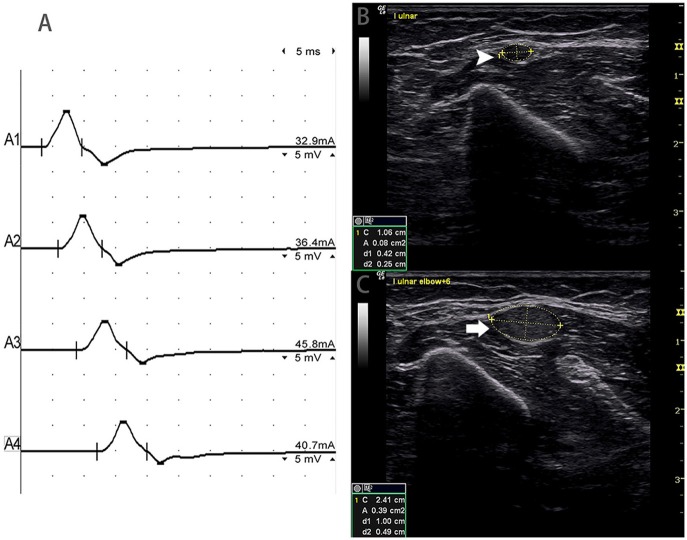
Mode 3. A 51-year-old woman with 4 years of left upper limb weakness and MRC grade 4 showed CSA enlargement without corresponding conduction block. (A) Standard segment motor nerve conduction study of the left ulnar nerve. No CB was detected. Nerve ultrasound study across the left upper arm of the ulnar nerve show: (B) CSA (white arrowhead) that was 8 mm^2^ at the site of A3 (latency 8.7 ms, duration 8.0 ms, amplitude 8.2 mv, area 19.2 mvms, conduction velocity 48.6 m/s) and (C) CSA (arrow) of the site 6 cm proximal to the elbow of the left ulnar that was 39 mm^2^ (latency 12.0 ms, duration 7.9 ms, amplitude 8.4 mv, area 19.5 mvms, conduction velocity 18.2 m/s), but no CB was detected across the same segment (A1, wrist; A2, below elbow; A3, above elbow; A4, axilla) (Video 3). CSA, cross-sectional area; CB, conduction block; l, left; r, right.
